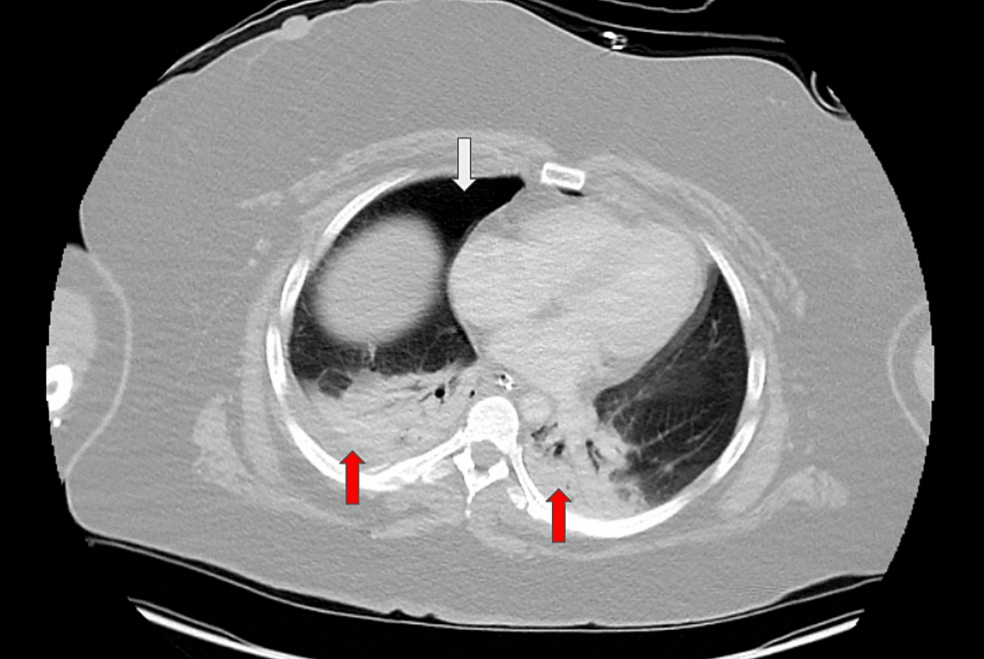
Axial CT with contrast shows right-sided pneumothorax (white arrow) and bilateral layering consolidations (red arrows).
